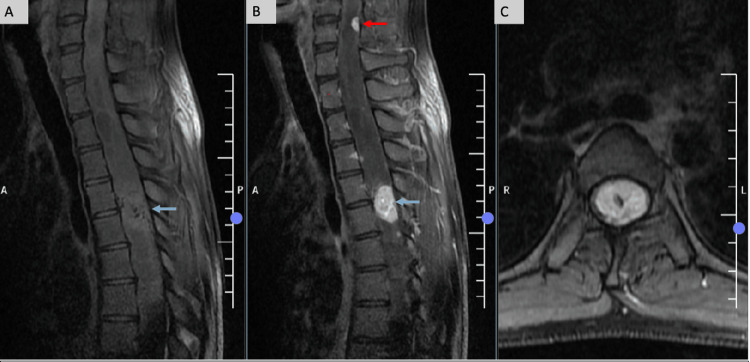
Pre- and post-contrast sagittal and axial T1-weighted image (A) Sagittal T1-weighted image showing an isointense intramedullary lesion at the D4-D5 level (blue arrow); (B) Avid, homogeneous post-contrast enhancement of the lesion (blue arrow) and an additional enhancing focus at the C5-C6 (red arrow); (C) Axial post-contrast T1-fat-suppressed image confirming the intramedullary location.
